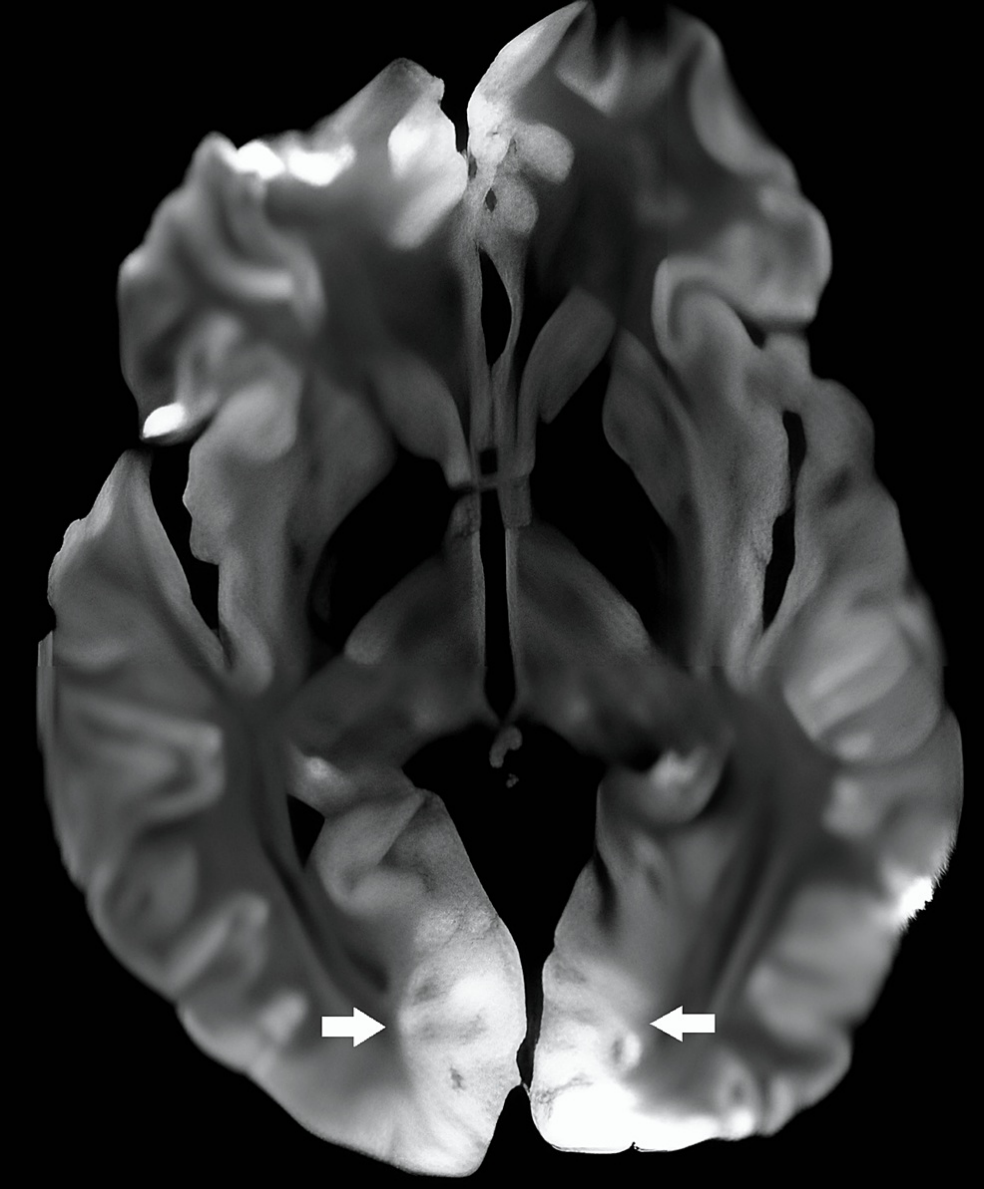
On the first postoperative day, brain diffusion MRI showed ischemia on both lateral occipital poles extending to the calcarine cortices (white arrows).
